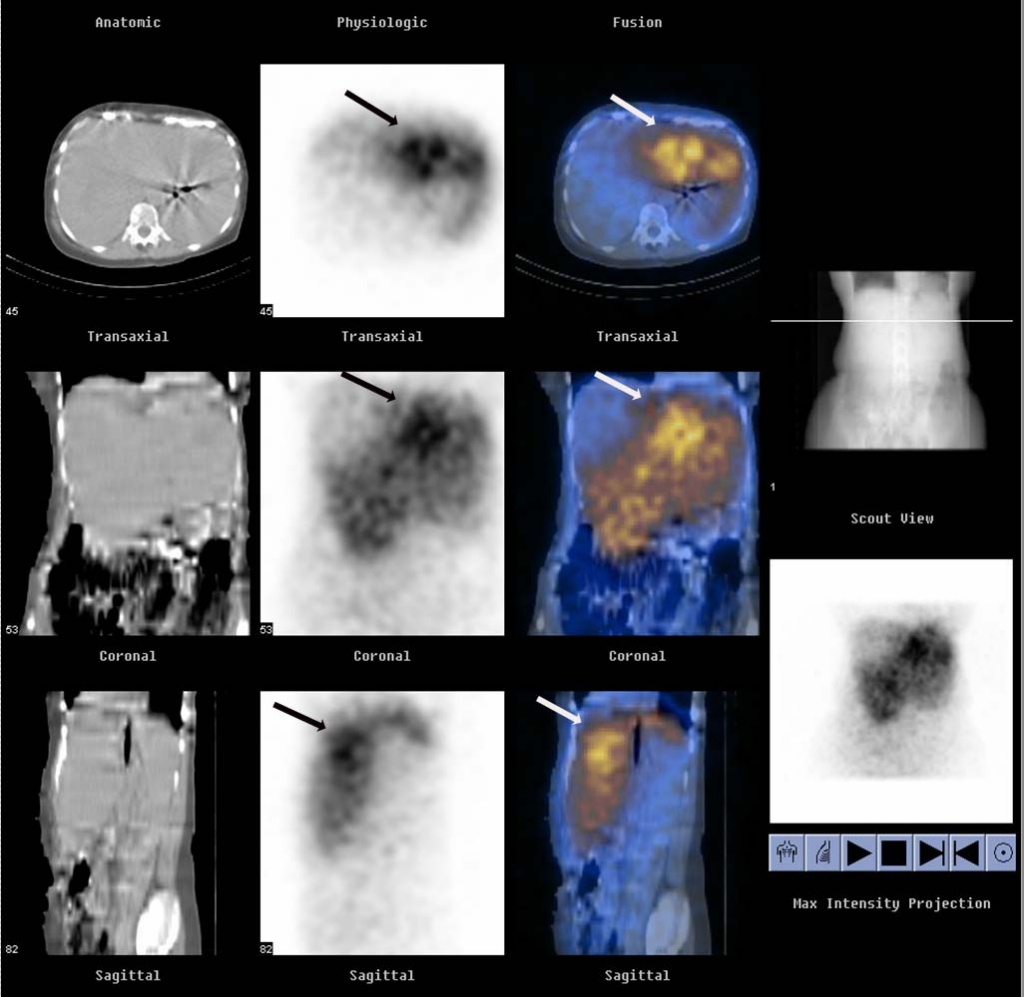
Bremsstrahlung imaging assists in confirming successful targeting of hepatic metastatic deposits from carcinoid tumour. Representative axial, coronal and sagittal CT images(first column), SPECT images (second column) and SPECT/CT fusion images (third column) obtained with a dual-modality imaging system) Hawkeye GE Medical Systems, Milwaukee, Wis) show selective activity in the left hepatic lobe (arrow) approximately 24 hours after intraarterial infusion of 90Y- bearing resin microspheres at a dose of 23.9 mCi (884.3 MBq).
